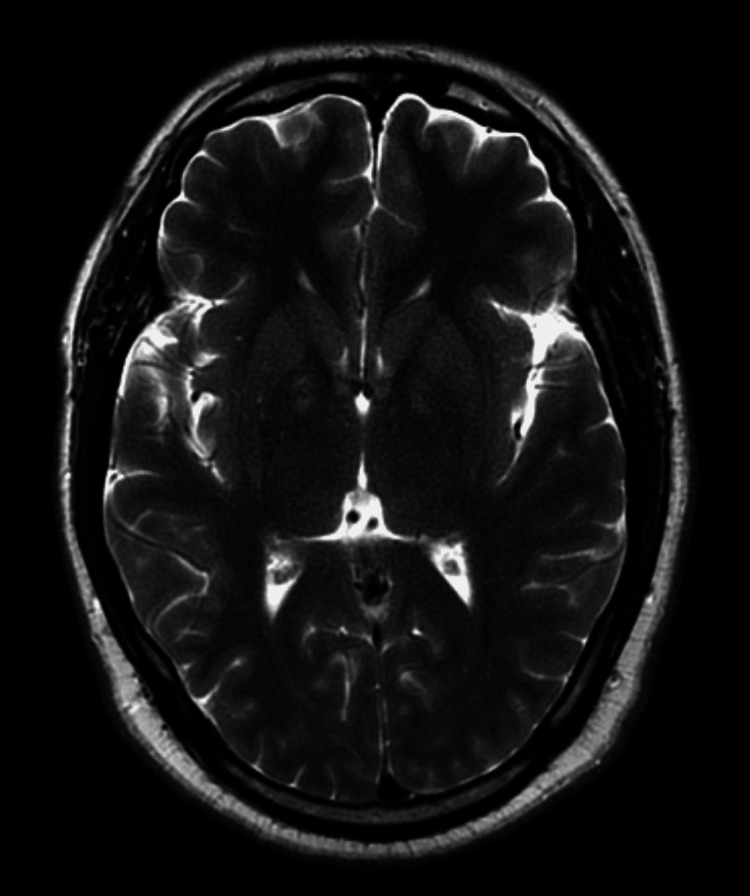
Contrast-enhanced MRI of the brain Contrast-enhanced MRI of the brain showing no evidence of intracranial pathology. There are no signal abnormalities or contrast-enhancing lesions in the temporal lobes. Ventricular size, sulcal pattern, and brain parenchyma appear within normal limits.
